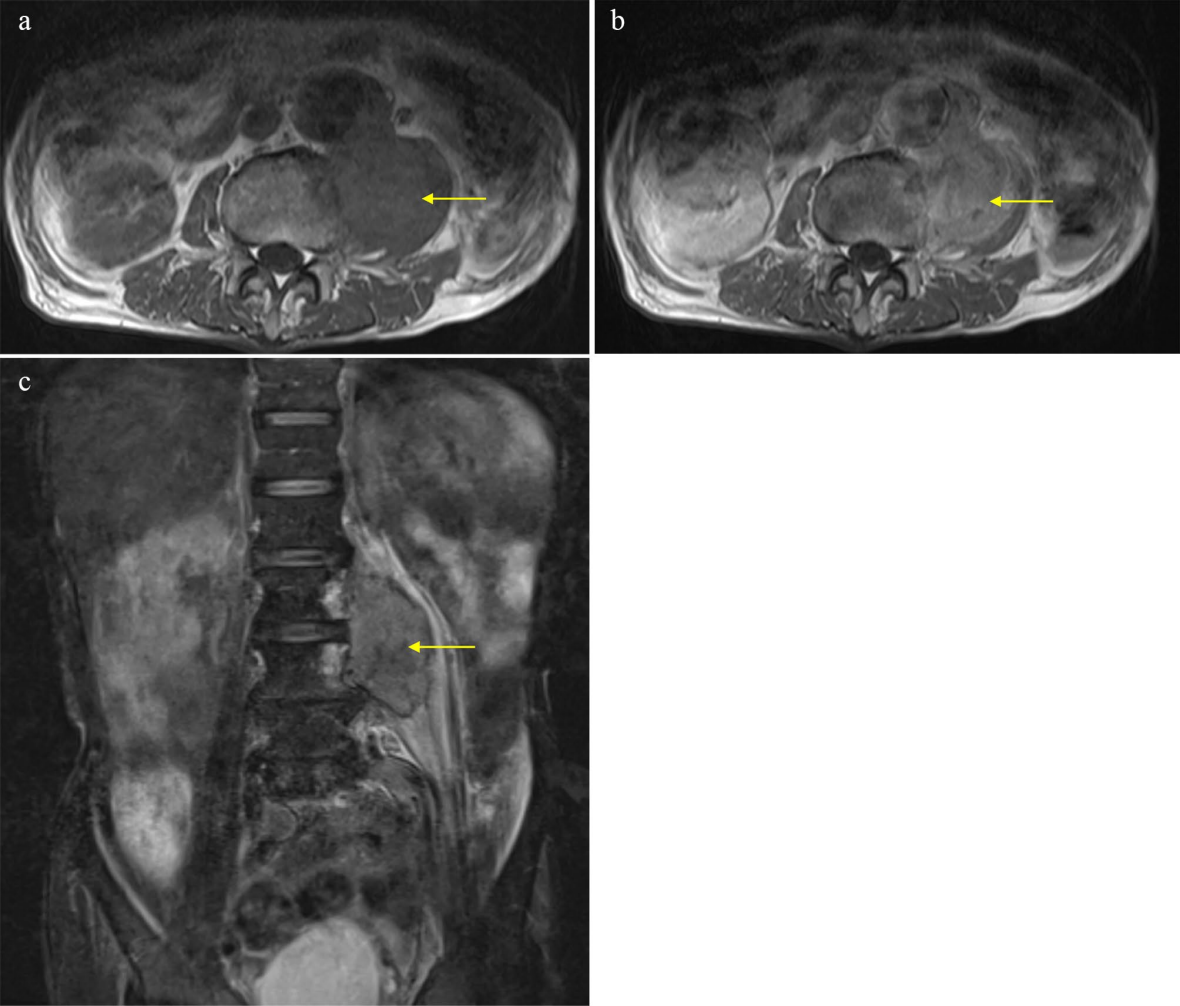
MRI results. (a) axial T1-weighted, homogeneous iso-intensities; (b) T2-fat suppression, heterogeneous high-intensities; (c) axial enhanced, heterogeneous-enhanced lesion
